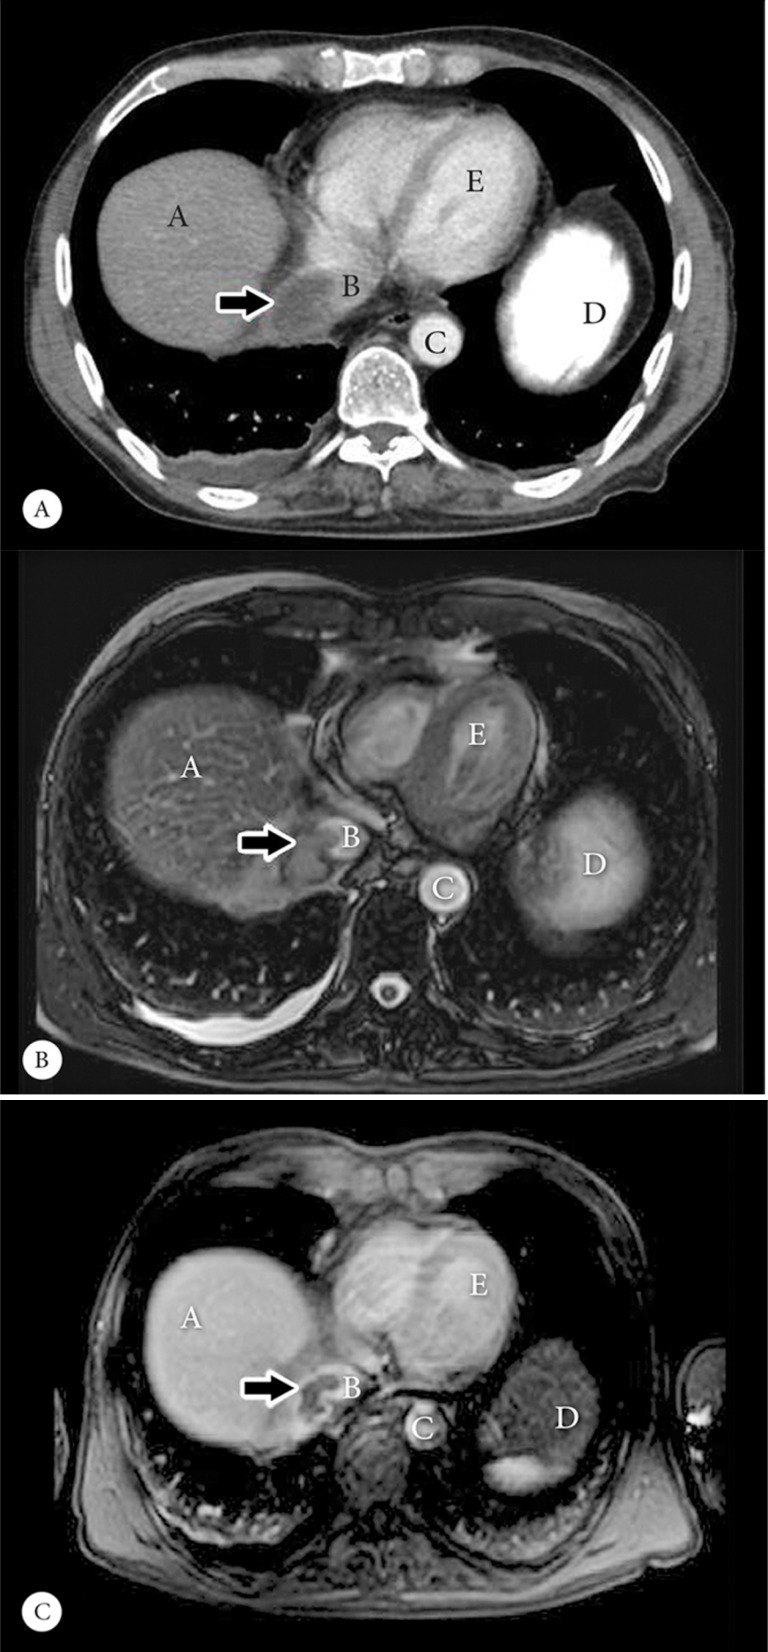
Abdominal contrast-enhanced computed tomographic axial scan (Panel A), showing a non-enhancing filling defect (arrow) in the upper portion of the inferior vena cava with extension to the right atrium. T2 weighted abdominal axial magnetic resonance imaging (MRI) (Panel B), showing a non-enhancing hyposignal filling defect (arrow) that is isosignal to the adjacent liver parenchyma. Contrast-enhanced T1 abdominal MRI with spoiled gradient echo (Panel C), showing a non-enhancing filling defect (arrow) as well as air in the biliary tract, which could be due to previous cholecystectomy and the anastomosis of the common bile duct to the small bowel.
